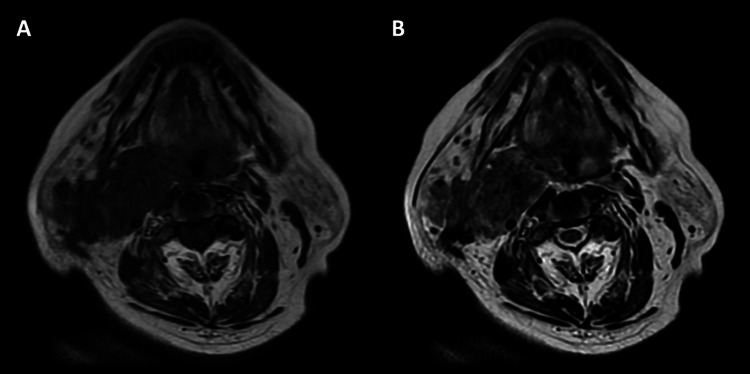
MRI of the neck. (A) and (B): Plain MRI: soft dense mass with indistinct borders in the right side of the neck, showing an intermediate signal on T1-weighted images and a heterogeneous low-to-moderate signal on T2-weighted images. MRI: magnetic resonance imaging.
